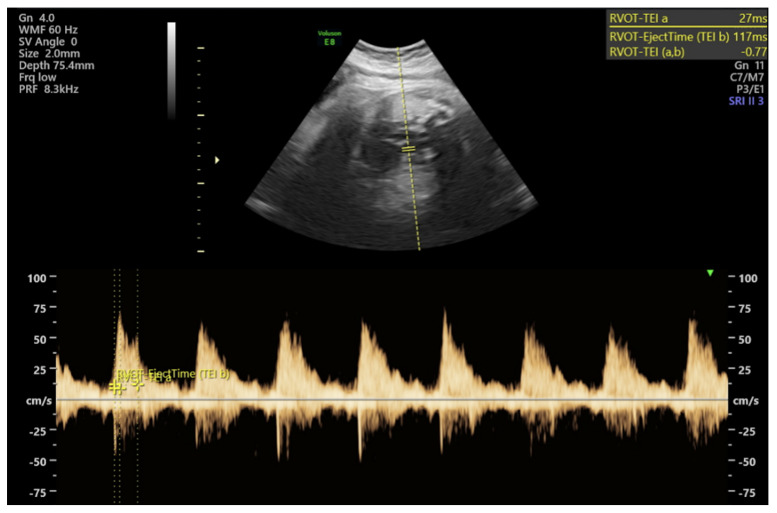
Fetal main pulmonary artery Doppler measurements.
